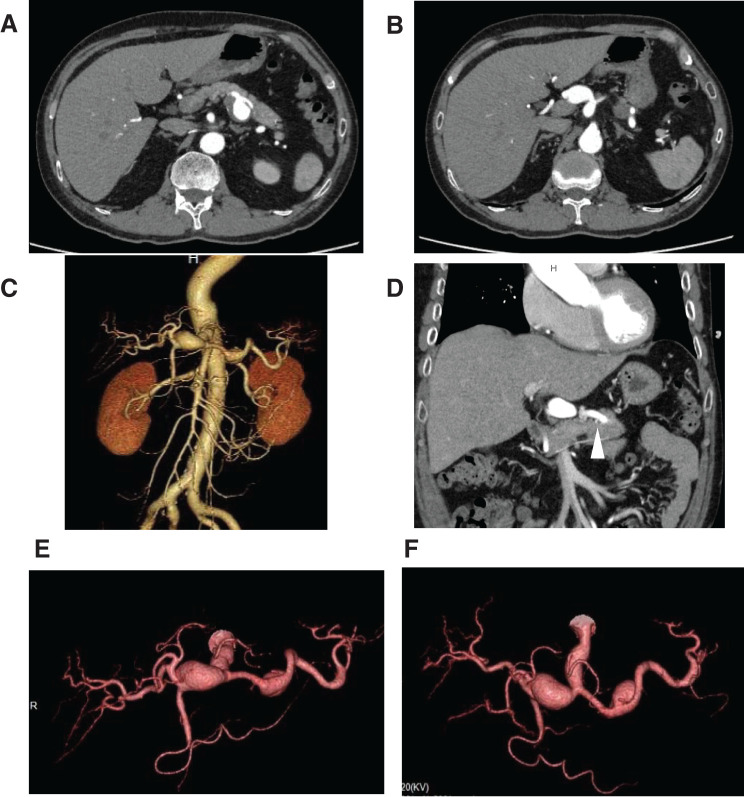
Fig. 1 CT and CT angiography. (A–C) CT angiography showed an SA aneurysm measuring 33 mm and a CHA aneurysm measuring 28 mm. The SMA was enlarged to 12 mm at the level of the jejunal branches, but no residual dissection was observed. (D) In the coronal view, the DPA branching near the aneurysm can be seen (arrowhead). (E) Left anterior oblique 9 and cranial 6 and (F) cranial 58. The CA had a normal diameter at the aortic bifurcation and at the branching points of the CHA and SA, but the CA was enlarged to 15 mm. CT: computed tomography; SA: splenic artery; CHA: common hepatic artery; SMA: superior mesenteric artery; CA: celiac artery; DPA: dorsal pancreatic artery
